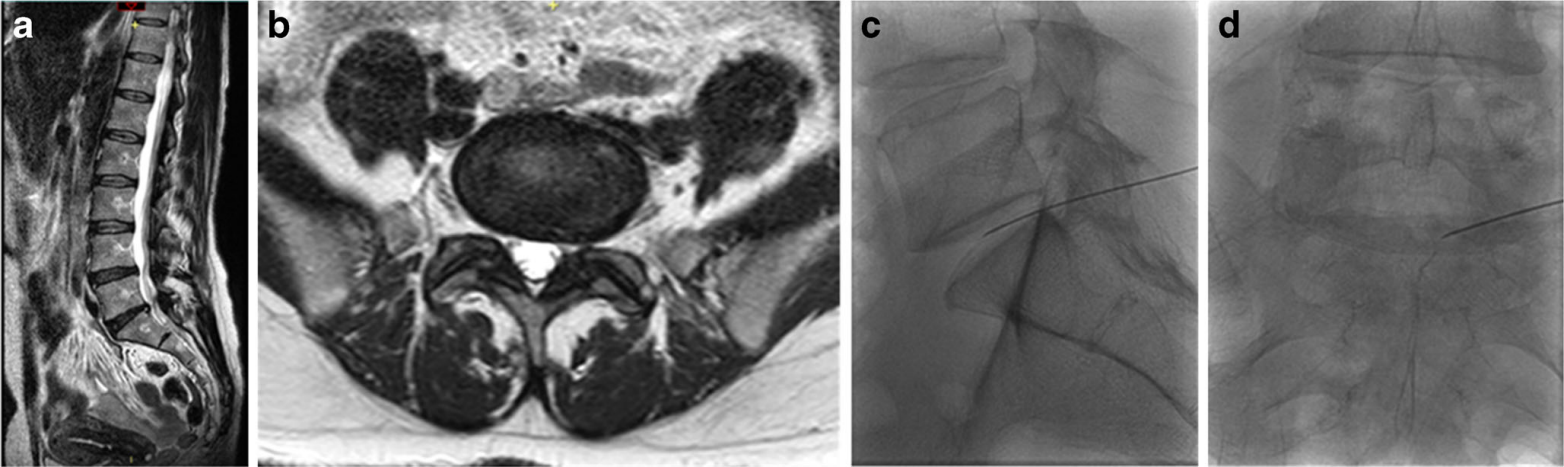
A 52-year-old women complaining of left lumbosciatica of 1-year duration and treated by intradiscal injection of an O_2_-O_3_ mixture. a MRI T2-weighted sagittal image, showing diffuse degenerative changes, with an associated bulging disc at L5-S1 level. b Axial MR T2-weighted image of the same patient shows diffuse disc bulge at the aforementioned level, more inclined to the left side with encroachment on the ipsilateral neural foramen. c, d Lateral and anteroposterior fluoroscopic images displaying the needle tip inside the disc center
